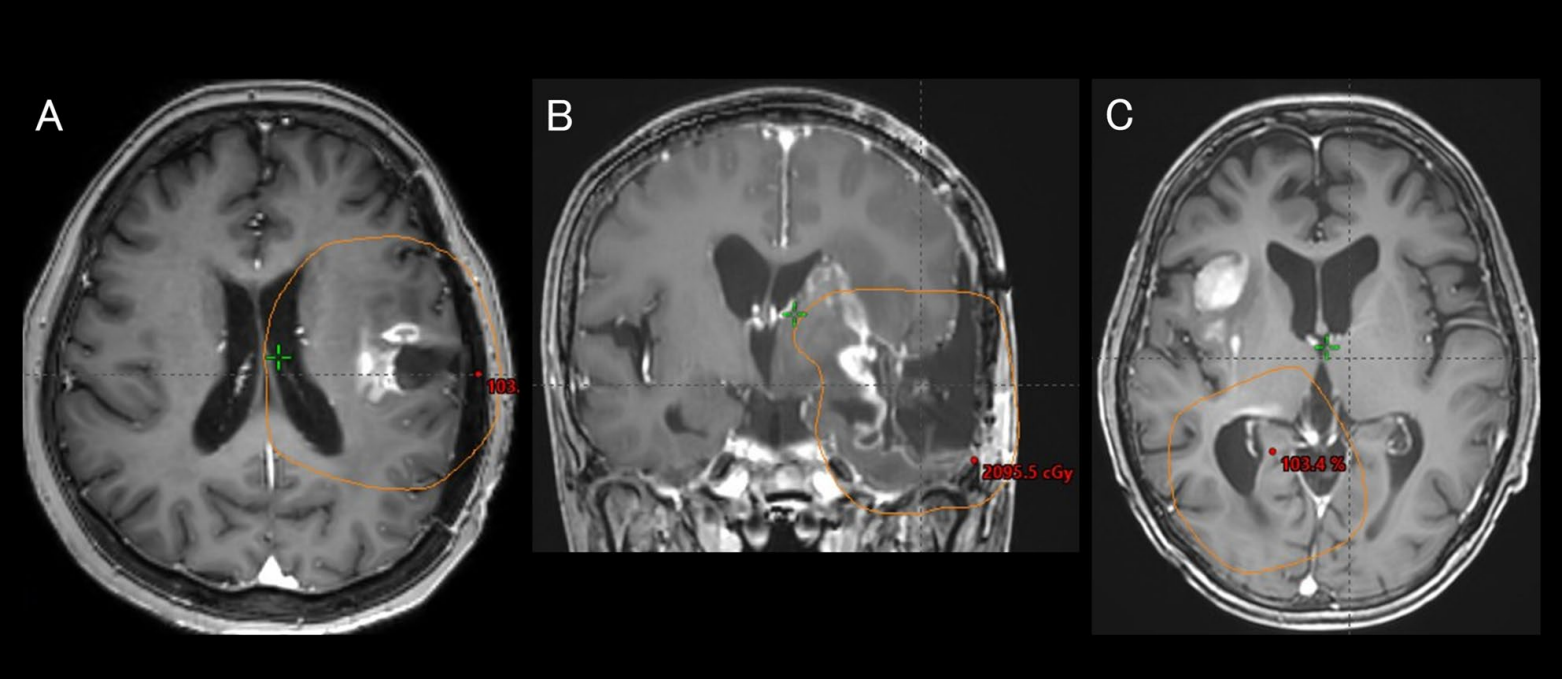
Recurrence patterns after adaptive radiation therapy. Magnetic resonance imaging images showing the recurrence patterns of glioblastoma. The orange line represents the 95% isodose line. (A) Central. (B) In-field. (C) Distant
